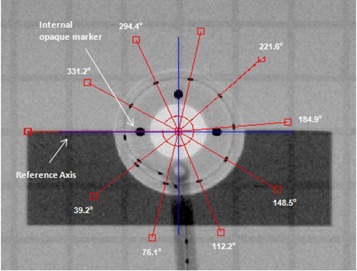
Reference radiograph with X‐ray marker showing angles measured with respect to reference axis (blue line) aligned with the four opaque markers.
